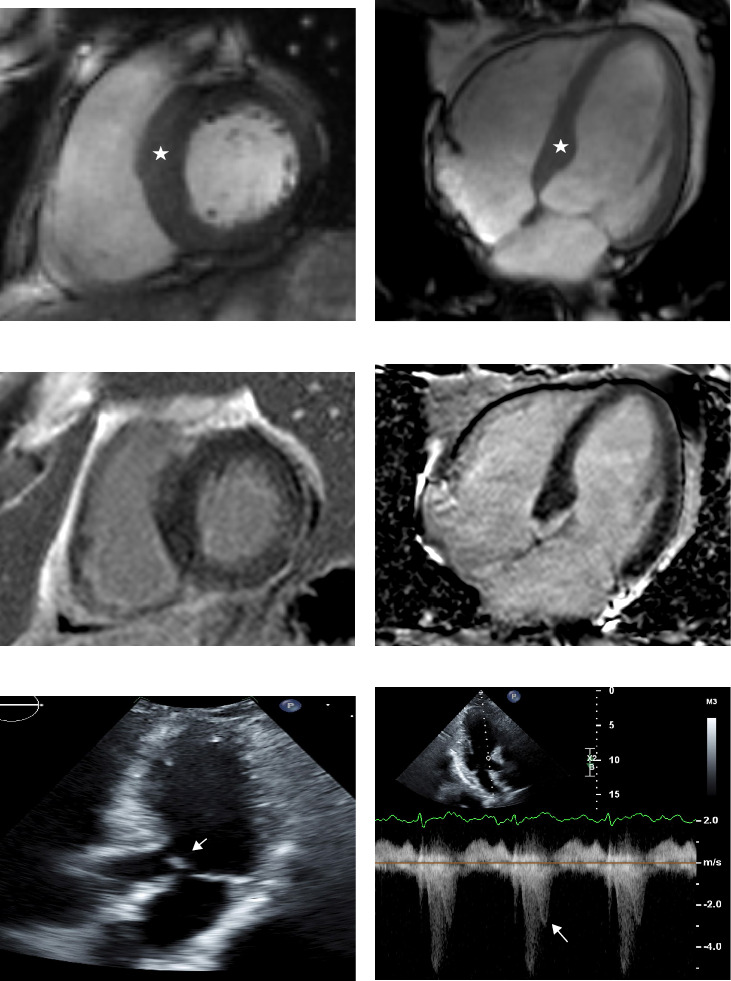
Cardiac magnetic resonance imaging and echocardiography. (A–D) Cardiac magnetic resonance imaging demonstrates asymmetric septal hypertrophy (star) (A–B) and absence of late gadolinium enhancement (C–D). (E) Transthoracic echocardiography demonstrates systolic anterior motion (SAM) of the mitral valve (arrow) with obstruction of the left ventricular outflow tract. (F) Continuous wave Doppler is consistent with dynamic left ventricular outflow tract obstruction due to SAM, with a late-peaking gradient of 37 mmHg (arrow).
